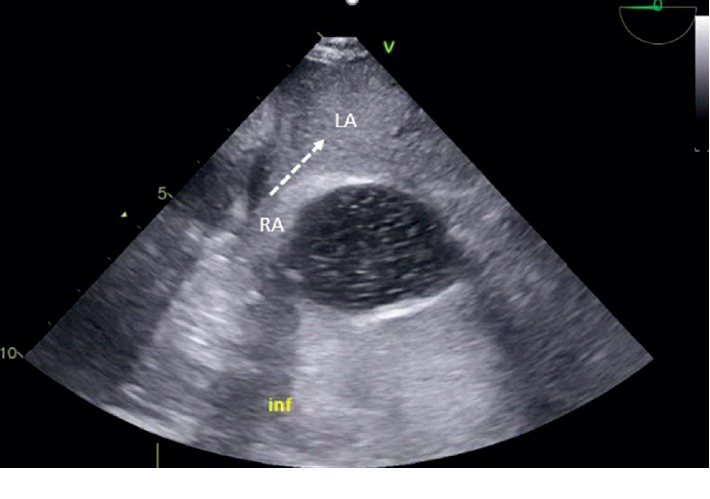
Transesophageal contrast echocardiogram: midesophageal aortic valve short axis view of atrial septal defect; inferior vena cava injection in the upright position with a massive right-left shunt. RA: right atrium, LA: left atrium.
